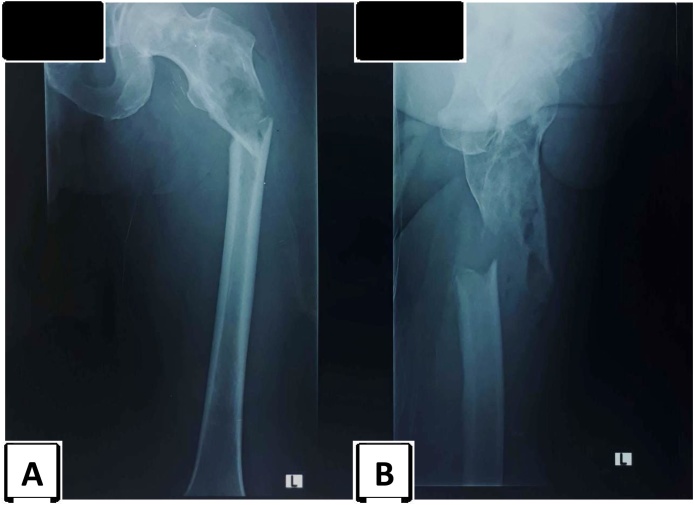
Radiographic pictures. Anteroposterior (A) and lateral (B) projections showed lytic lesion in the area of subtrochanter of the left femur with pathological fracture and soft tissue swelling.
